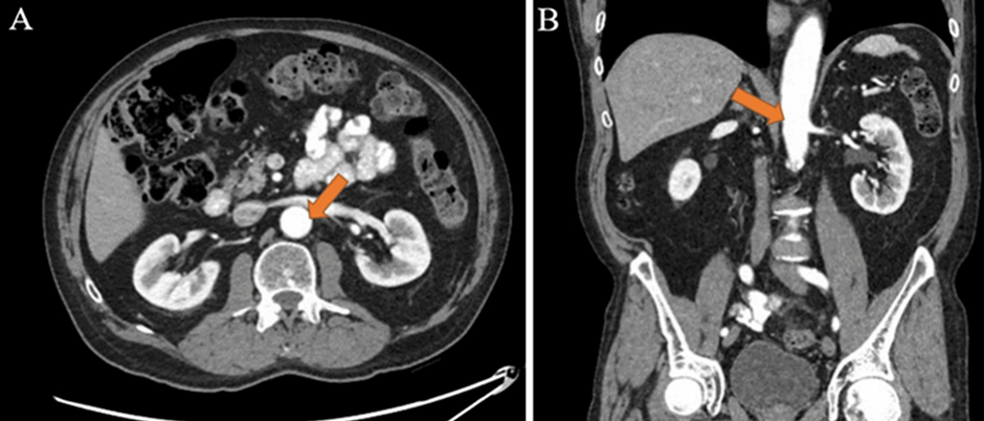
CT of the abdomen/pelvis with no aortic thrombosis present (as shown by the arrows) six weeks prior to the initiation of capecitabine-oxaliplatin chemotherapy. A: Axial view. B: Sagittal view.
